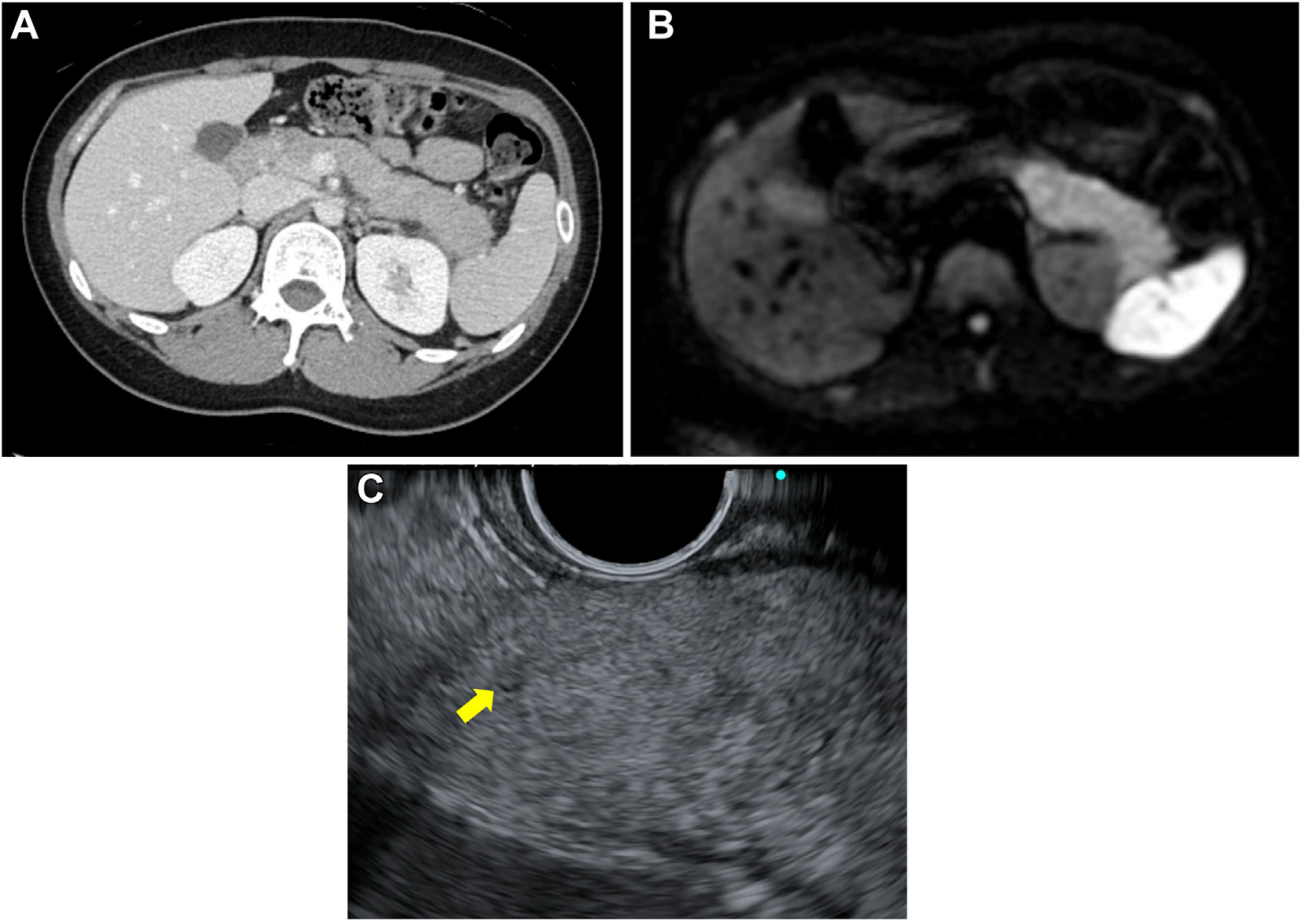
Various images with findings of the pancreatic body and tail at the initial visit. (A) Contrast-enhanced computed tomography shows swelling, with heterogeneous poor contrast. (B) DWI shows a high-intensity area. (C) EUS shows slight swelling and high-echo parenchyma. Yellow arrow shows an edematous MPD.
